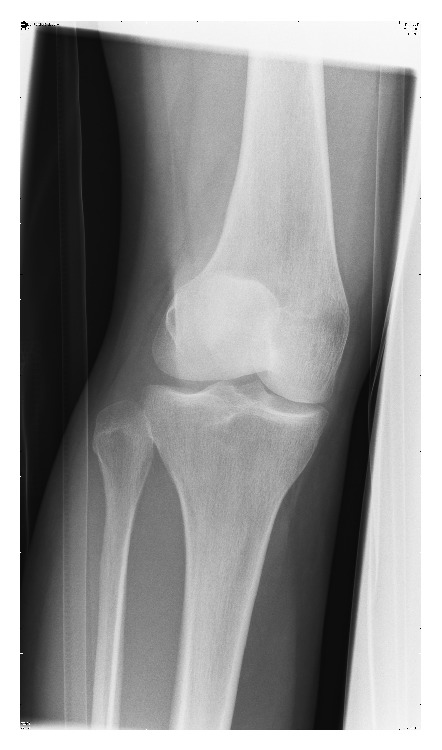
Anterior-posterior radiograph of the knee with a laterally dislocated patella.
